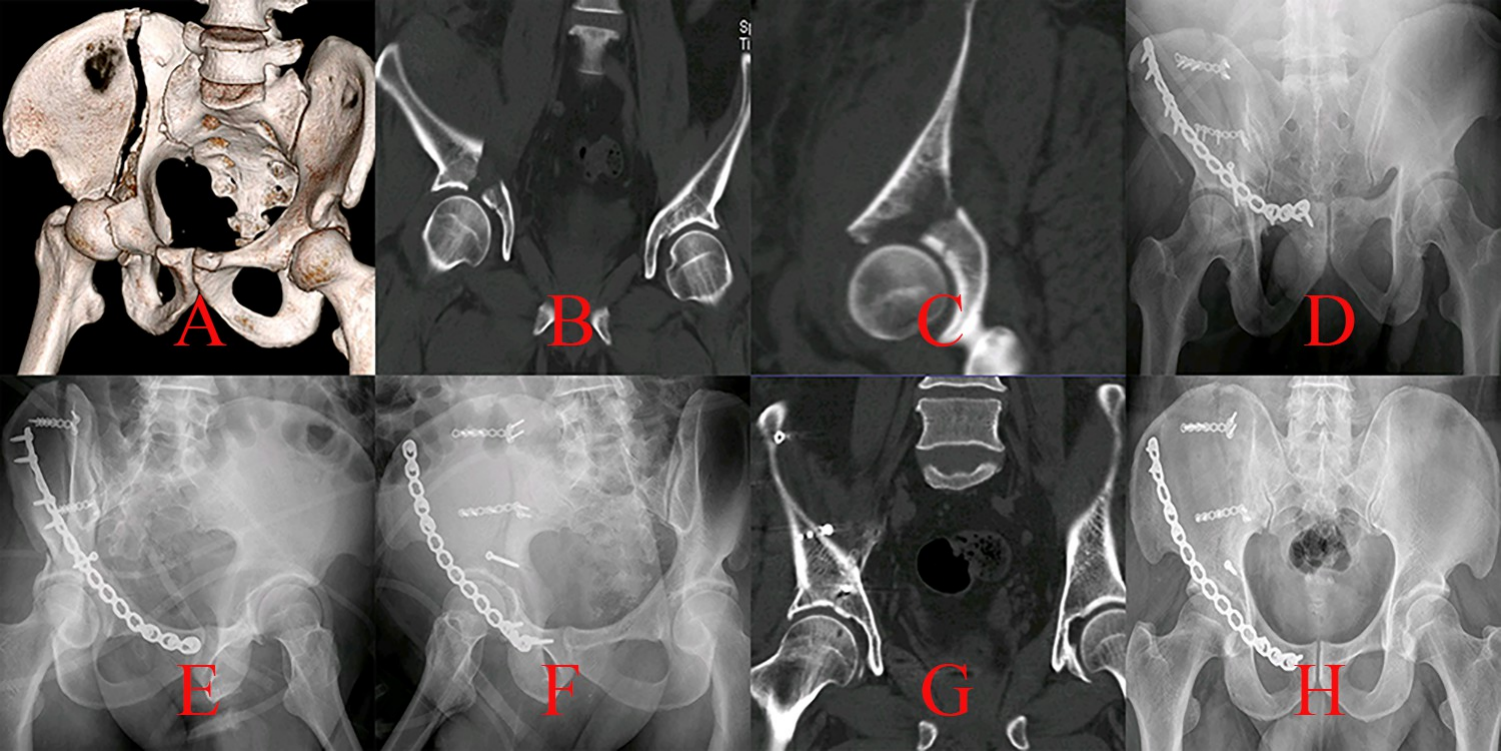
A case with A3.2 injury managed through the ilioinguinal technique was presented. A, cephalad and separated detachment of roof column on 3D reconstruction; B-C, cephalad and separated detachment lead to mismatch of acetabulum and femoral head on preoperative CT scans; D-F, the reconstructed acetabulum presented a larger diameter than the corresponding femoral heads and the contralateral acetabulum on postoperative images (imperfect reduction quality), an additional lag screw was placed to enhance the stability of roof articular surface; G, postoperative CT scan; H, AP view 3 months postoperatively.
